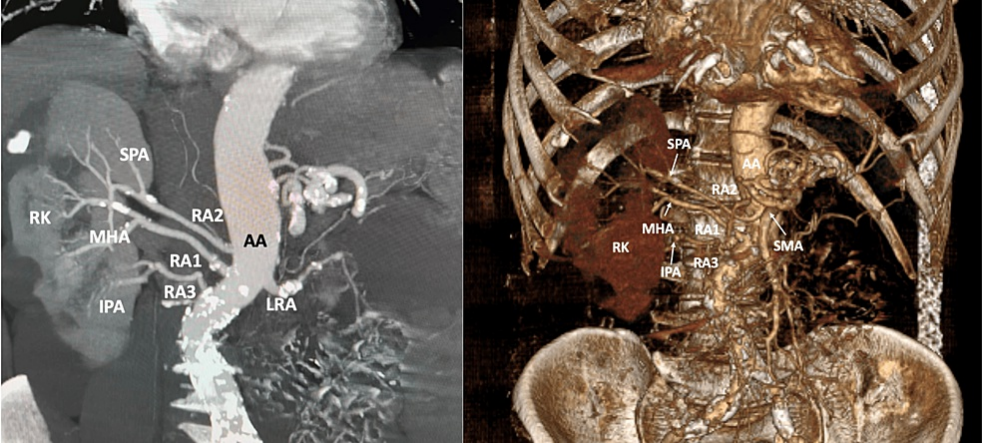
The right renal variant vasculature was identified in CTA before and after 3D reconstruction. Three renal arteries (RA1, RA2, and RA3) originated from the AA. After observing their course in the kidney, these arteries were characterized as the MHA, SPA, and IPA. CTA: computed tomography angiography, AA: abdominal aorta, MHA: main hilar artery, SPA: superior polar artery, IPA: inferior polar artery, RK: right kidney, SMA: superior mesenteric artery, LRA: left renal artery
